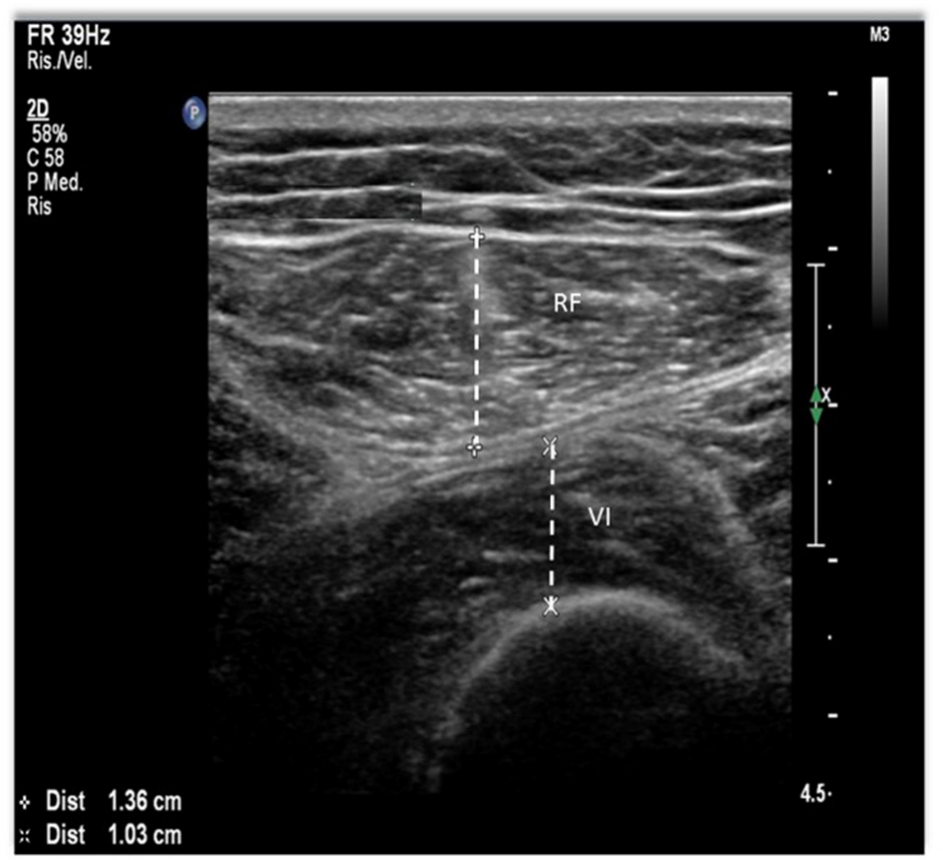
Quadriceps muscle ultrasound (US) methodology. The points of interest correspond to the midpoint and the lower third between the anterior superior iliac spine (ASIS) and the upper pole of the patella. Using a B-mode ultrasound with a linear transducer, we obtain the image on the right. Rectus femoris (RF) and vastus intermedius (VI) thickness, a measure on the inner edge of the muscle.
